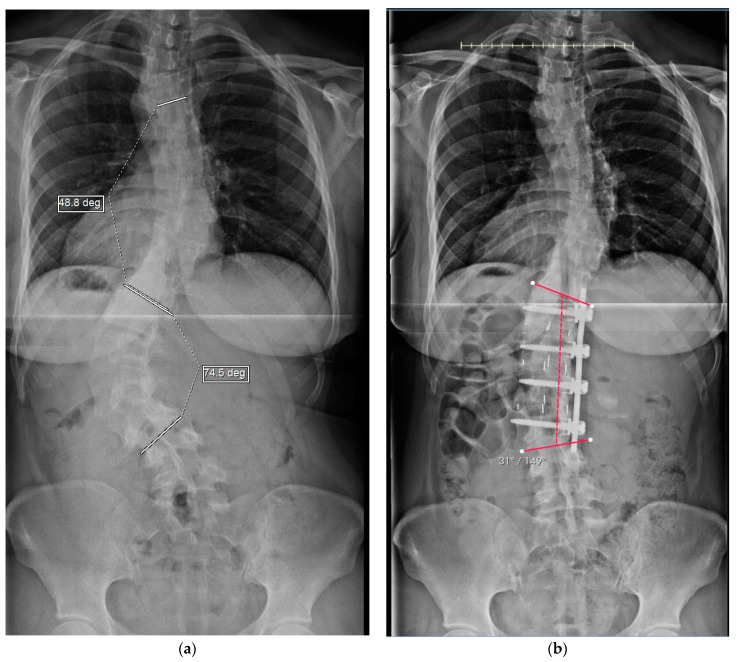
Preoperative and postoperative coronal radiographs (a,b).
